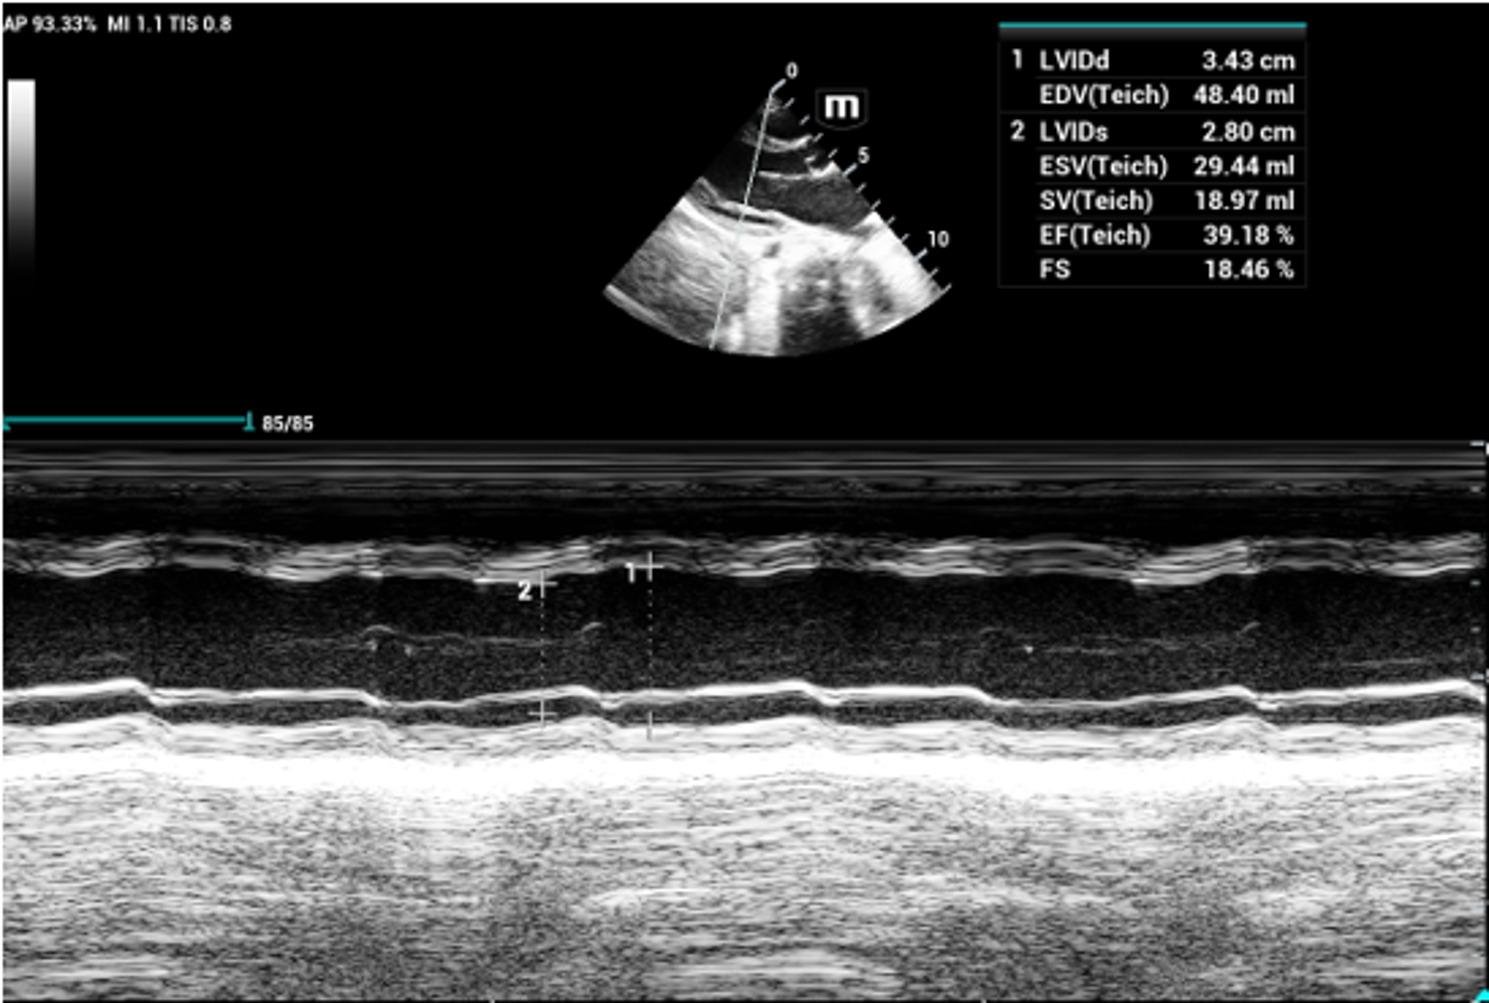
Transthoracic echocardiography with M-mode: showing of impaired left ventricular systolic function
